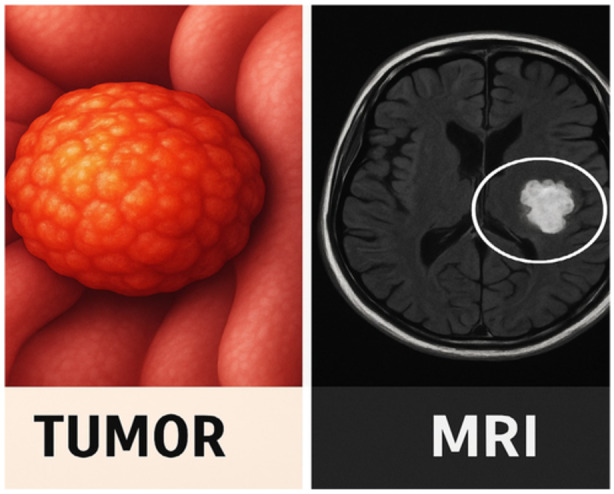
Visual of tumor cell and MRI image.
